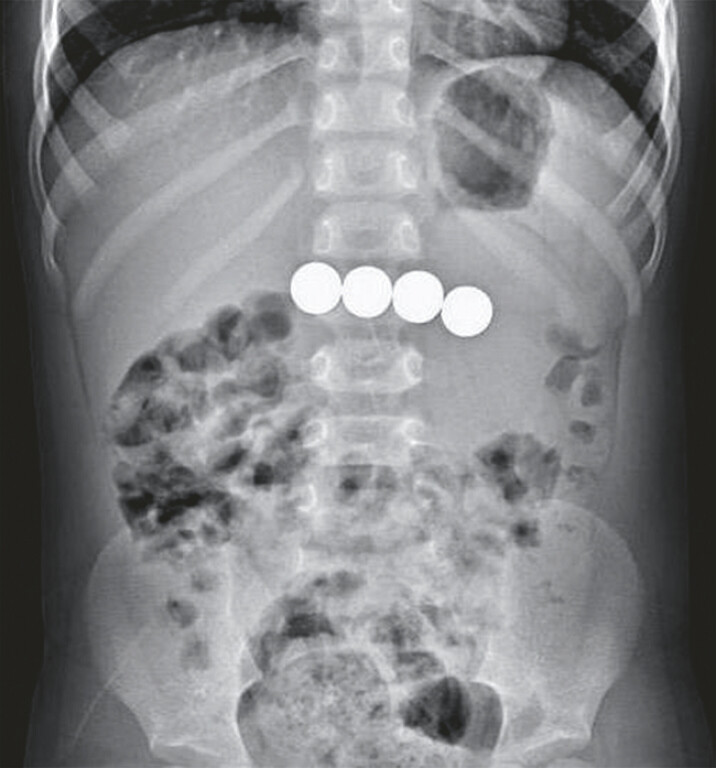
An abdominal X-ray reveals the presence of four spherical foreign bodies retained in the upper abdomen, with no evidence of pneumoperitoneum.
